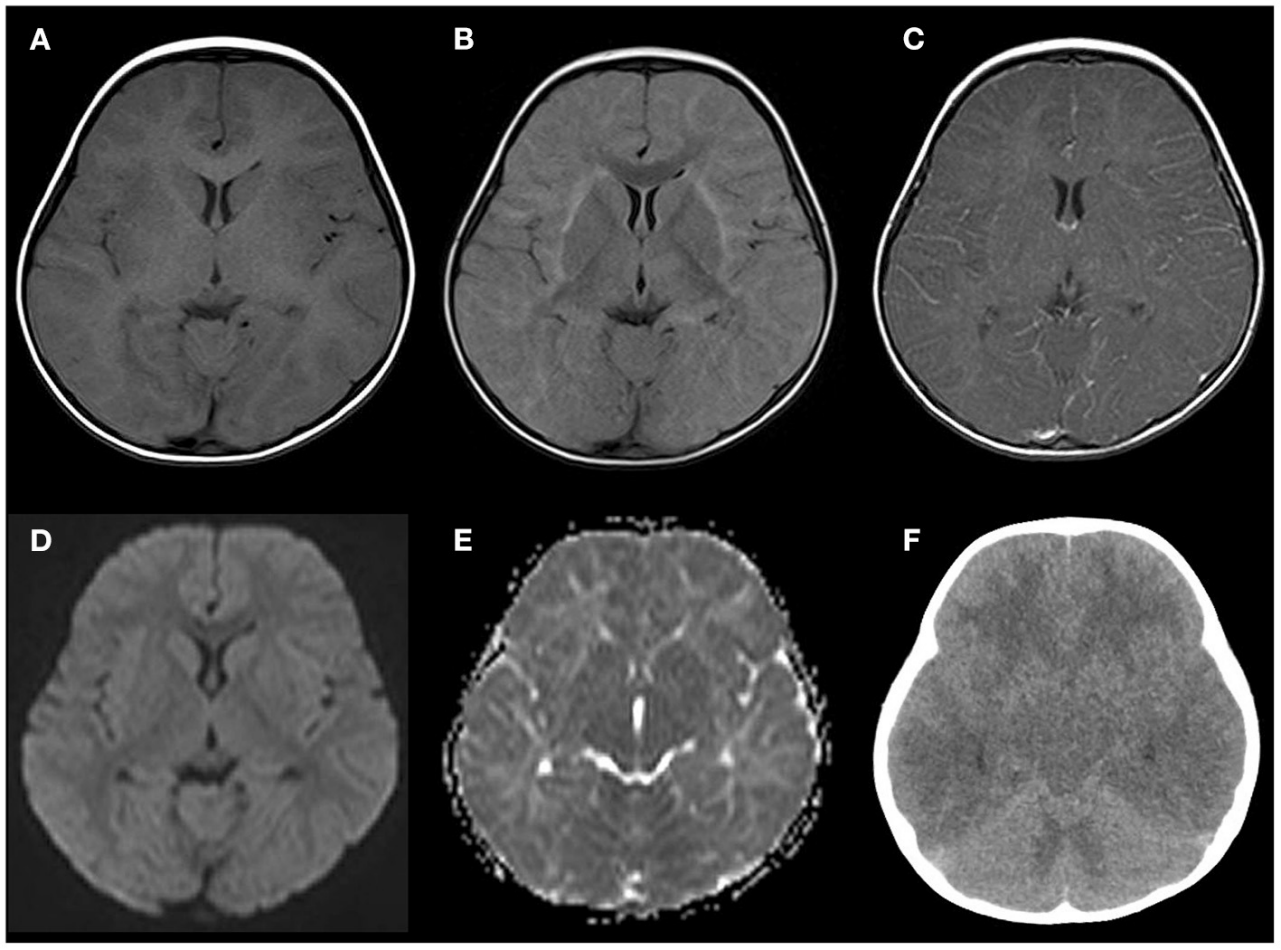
Neuroimaging findings on days 13–14. (A–E) Several sequences of the head MRI on day 13. (A) Isointense white matter lesions on a T1-weighted image (T1WI). (B) Fluid-attenuated inversion recovery image showing a high intensity in the subcortical white matter and external capsule. (C) Post-gadolinium T1WI showing no enhancement in the white matter or perivascular lesions. (D) Diffusion-weighted imaging showing isointense signals in the white matter lesions. (E) An apparent diffusion coefficient map showing diffuse hyperintensity in the white matter. (F) Brain computed tomography (CT) performed 8 h after the MRI demonstrating cerebral and cerebellar edema with sulcal and cisternal effacement.
